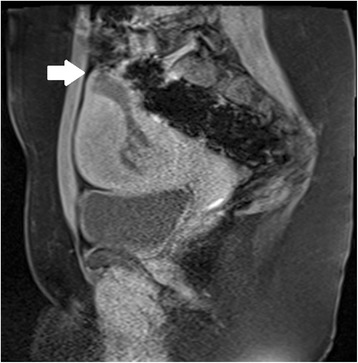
Interstitial ectopic pregnancy by magnetic resonance imaging, T1 weighted. The arrow indicates thin (<5 mm) myometrium overlying the ectopic pregnancy. In a stable patient, MRI may be useful in the confirmation of interstitial pregnancy location
